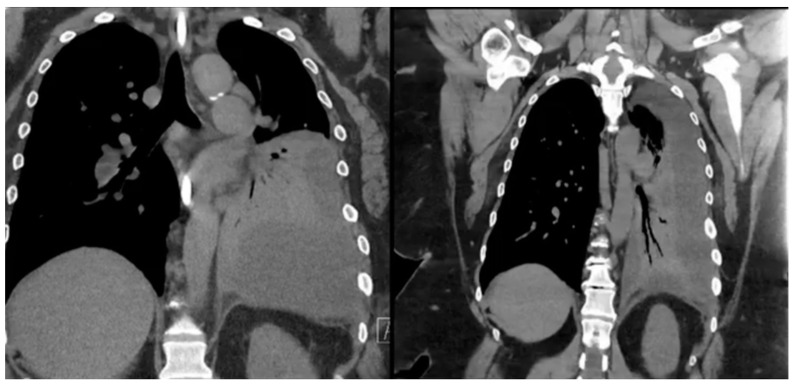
Chest computed tomography (CT) scan showing obliteration of the left lung space in a patient with gastro-pleural (GP) fistula.
